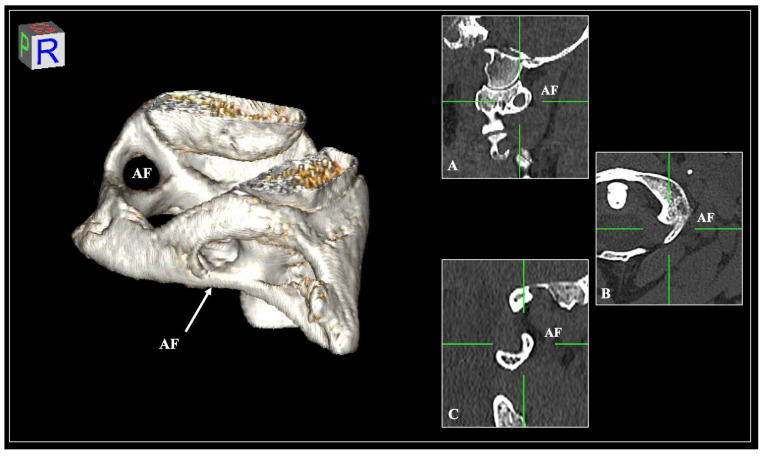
The arcuate foramen (AF) is depicted in a computed tomography scan. The AF is well-represented in sagittal slices (A) but not in axial and coronal slices (B,C).
